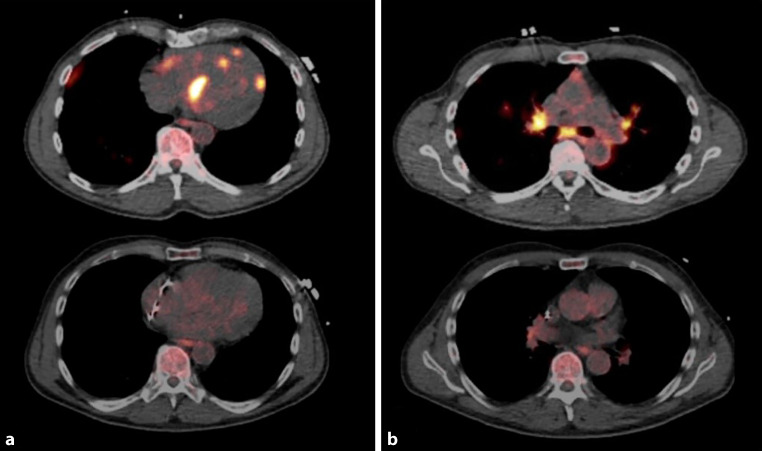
a Increased metabolic activity in PET/CT imaging with 332 MBq (F-18)-fluorodeoxyglucose in the left ventricular myocardium mainly basal anteroseptal, midventricular dorsal and the inferior papillary muscle. Right ventricular involvement is low (*top*). Remission after 5 weeks follow-up under immunosuppressive monotherapy with 50 mg prednisolone per day (*bottom*). b Bipulmonary increased metabolic activity mainly in the middle lobe and right upper lobe and additionally prominent mediastinal lymph nodes (*top*). Remission of metabolic activity due to immunosuppressive therapy (*bottom*)
